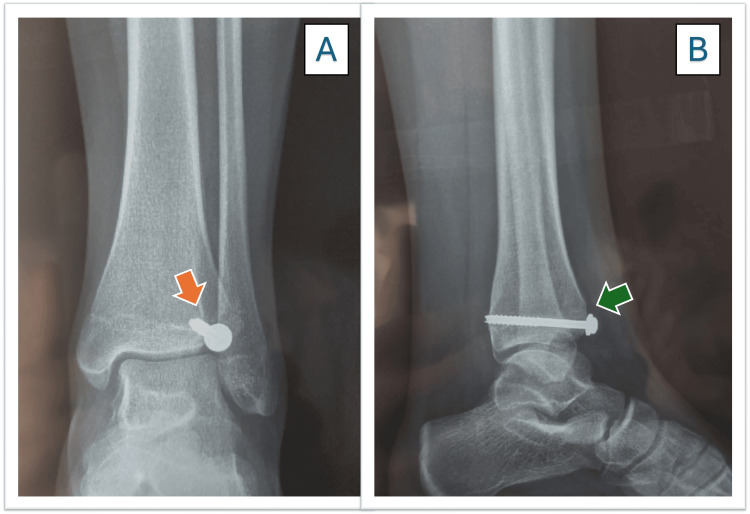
Anteroposterior and lateral view radiographs three months after surgery. A) The orange arrow shows a correct consolidation in the anterior-posterior view. B) The green arrow shows a correct consolidation in the lateral view.
